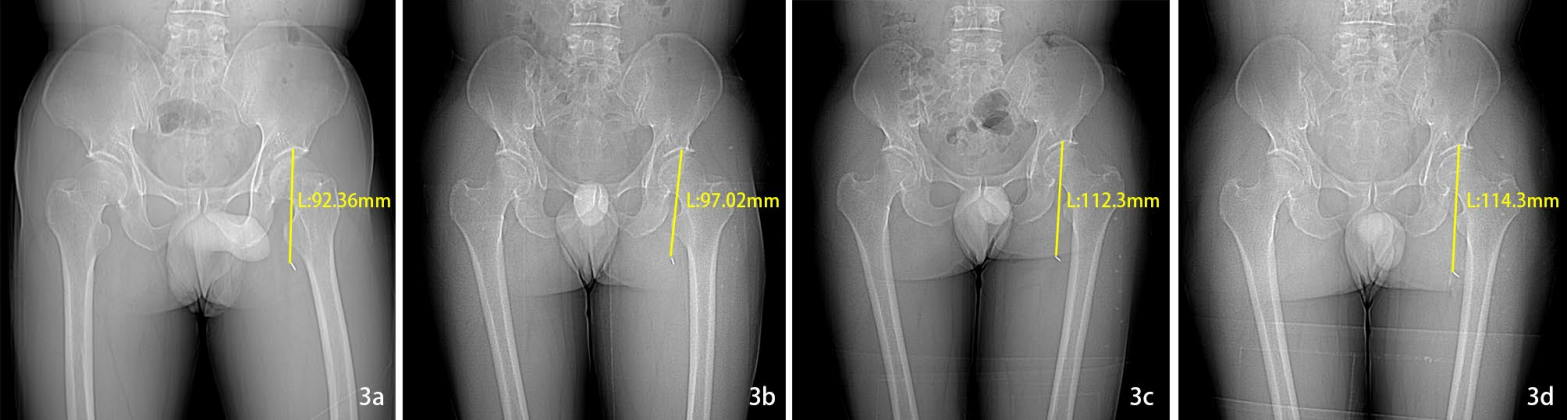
The X-ray showed the different location of the metal foreign body at different times (anteroposterior position)
